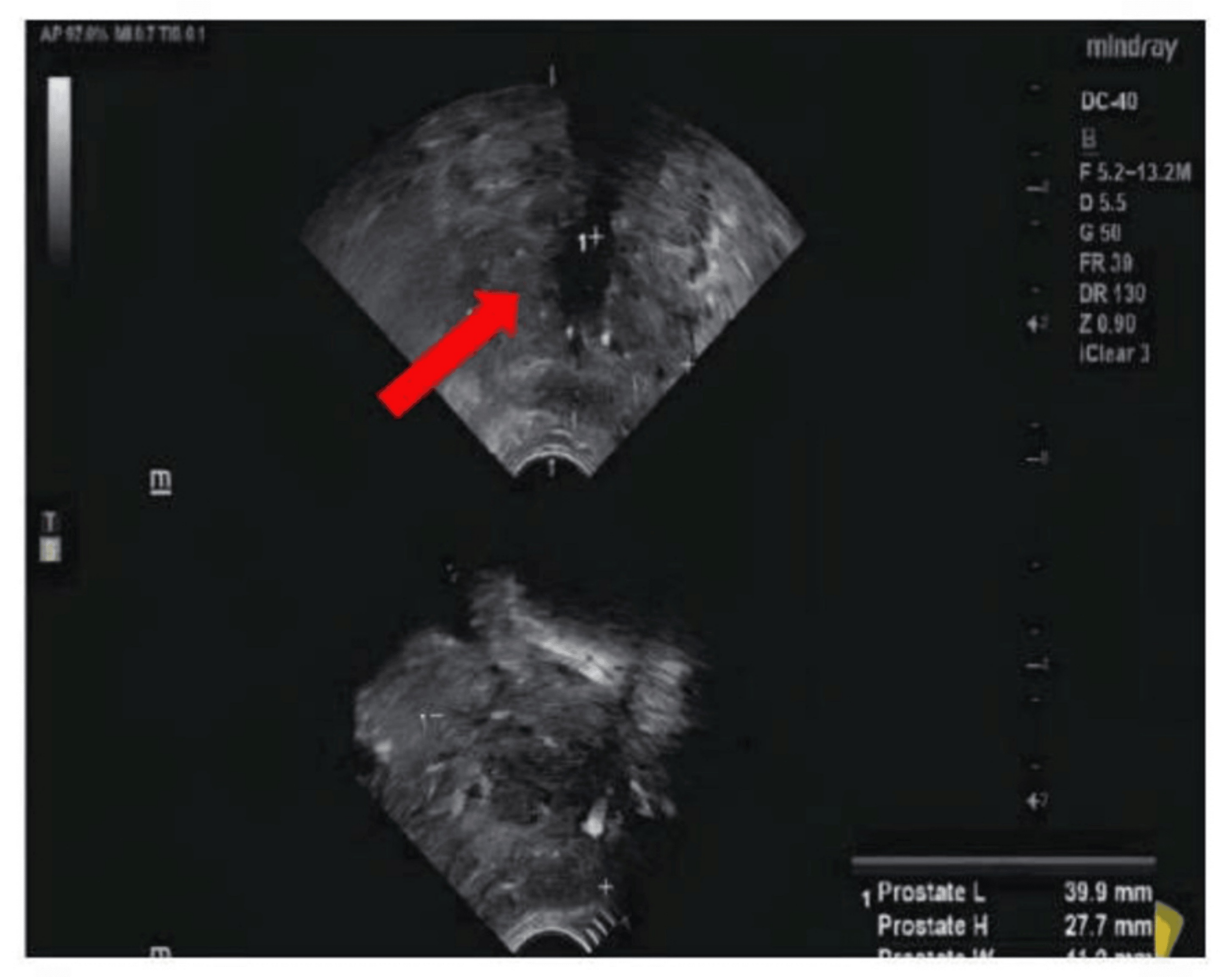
Transrectal ultrasound-guided prostate biopsy (arrow).
